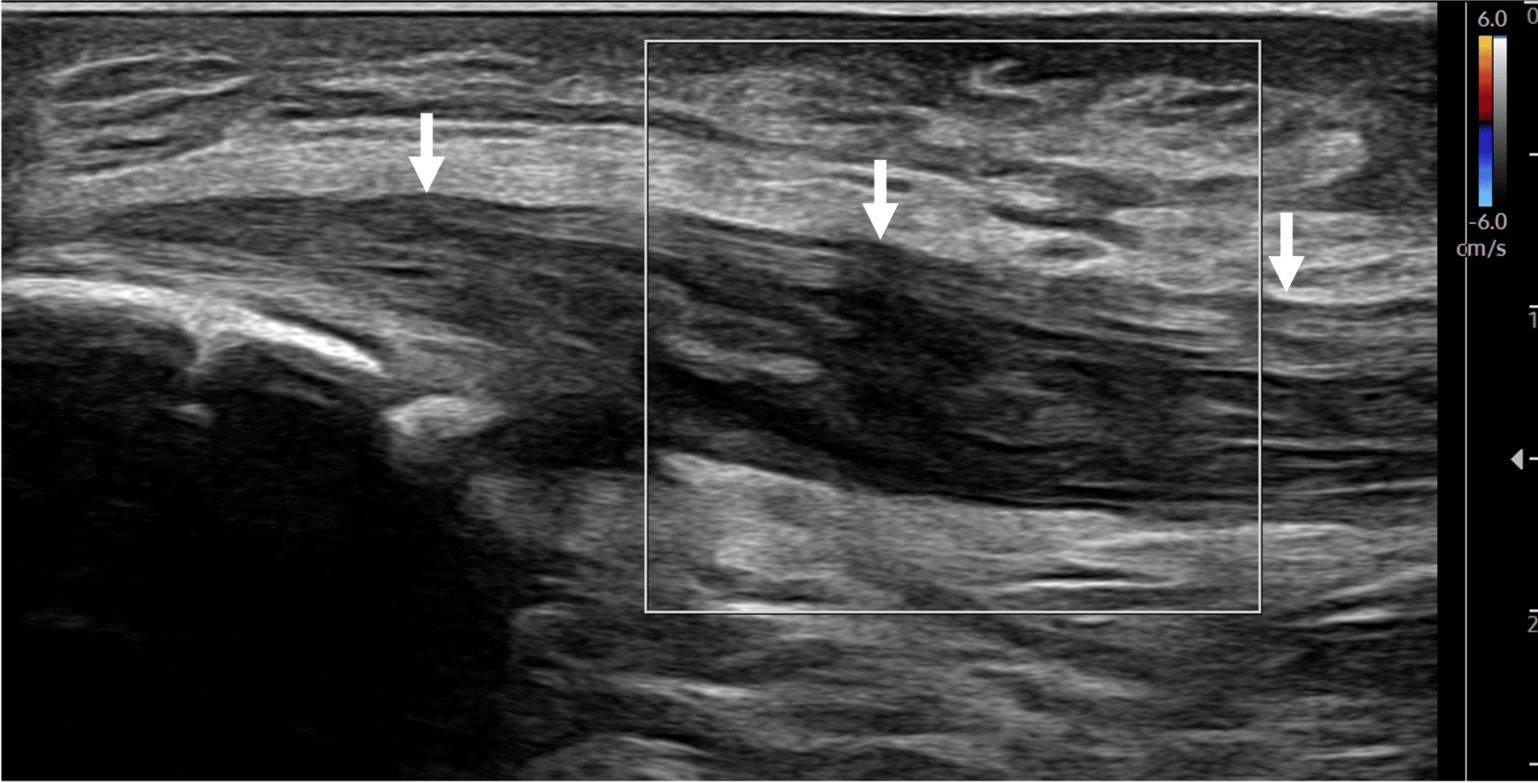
Tendinosis of the proximal patellar tendon in a 22-year-old man. Long-axis view of the patellar tendon with Color Doppler tool showing no hyperemia within the tendon Arrows: Patellar tendon.
